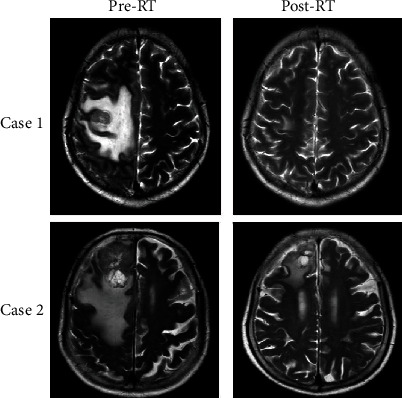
The MR images of 2 patients who underwent FSRT at pretreatment and 2 months posttreatment. Complete and partial response was found in case 1 and case 2, respectively. Case 1 is a case of lung cancer with right frontal lobe metastases which achieved complete remission in 2 months after receiving 7 Gy/F × 5F radiotherapy, whereas case 2 is a case of breast cancer with right frontal lobe metastases which achieved partial remission after 5 Gy/F × 5F radiotherapy.
